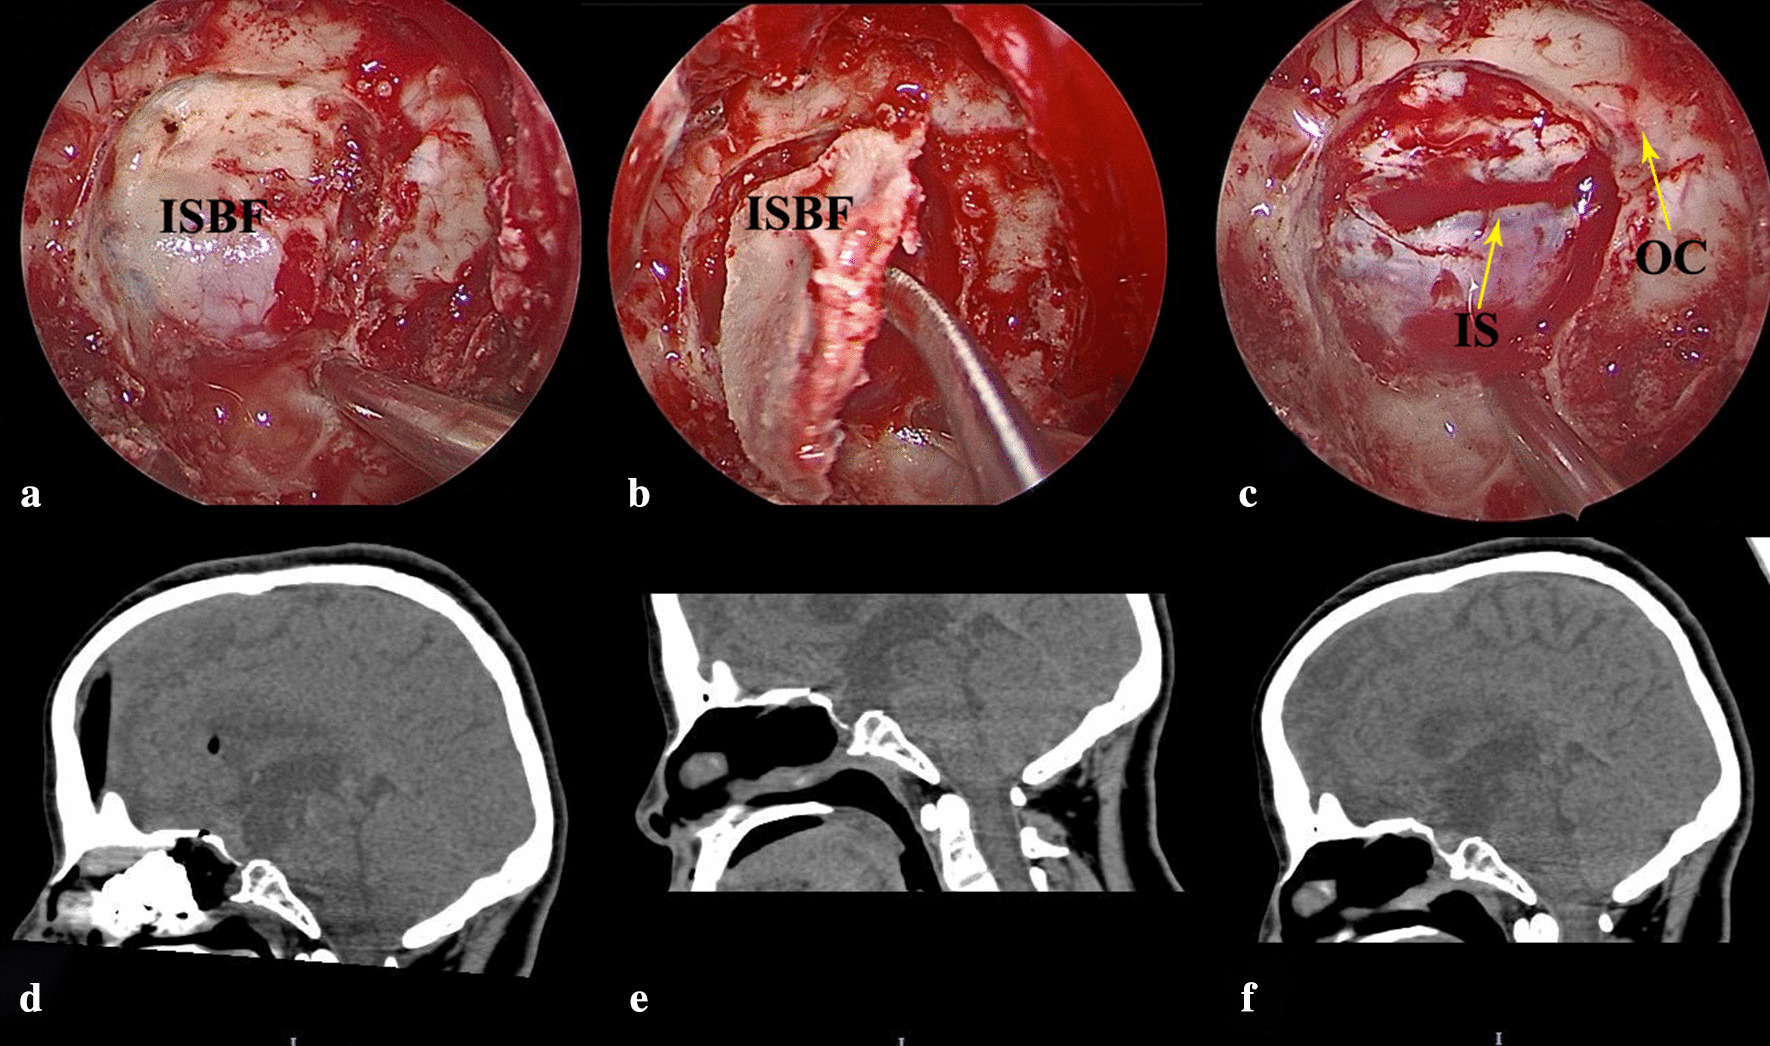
The intraoperative photographs show the harvesting of the “In Situ Bone Flap” and the evolution of the skull base defect postoperative healing. a The dimension of the designed ISBF ranged from one lateral cavernous sinus to the other, and from the planum sphenoidale to the sellar floor; b The elevation of the ISBF from the dura below it using a Cottle dissector; c The endoscopic view shows the intact dura, intercavernous sinus (IS) and optic canal (OC) after removing the In Situ Bone Flap. d–f The postoperative sagittal CT images shows the process of healing of the In Situ Bone Flap, at one day (d), 3 months (e) and 6 months (f), postoperatively
